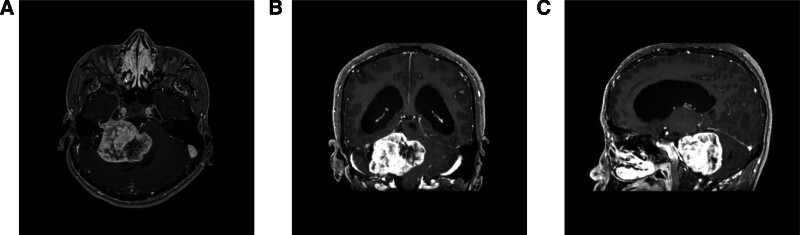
Preoperative CE-MRI. (A–C) Axial, coronal, and sagittal views of the tumor, respectively. CE-MRI = contrast-enhanced magnetic resonance imaging.
